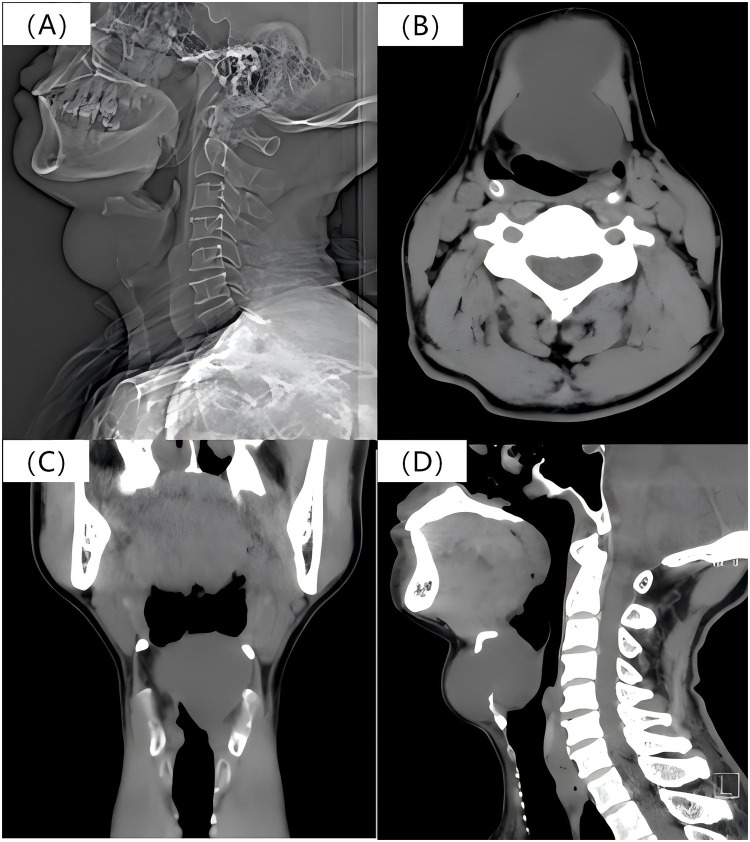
Preoperative computed tomography findings of the giant infrahyoid thyroglossal duct cyst. (A) Lateral scout view showing a large soft-tissue mass in the anterior neck. (B) Axial CT image showing a cystic low-density lesion inferior to the hyoid bone, with compression of the adjacent laryngeal cavity. (C) Coronal CT image showing the midline location of the lesion in the infrahyoid neck. (D) Sagittal CT image showing a large infrahyoid cystic lesion extending deeply and causing secondary compression and narrowing of the adjacent laryngeal cavity.
